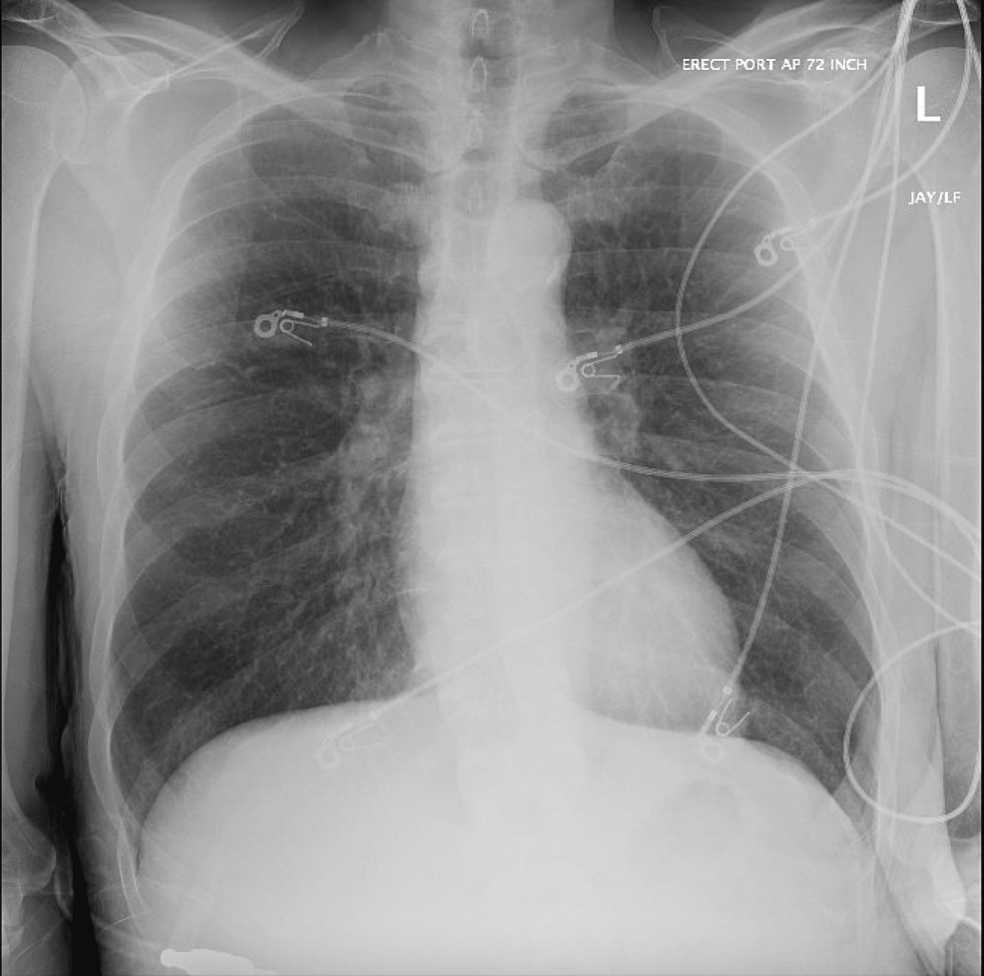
Chest radiograph six months after discharge
